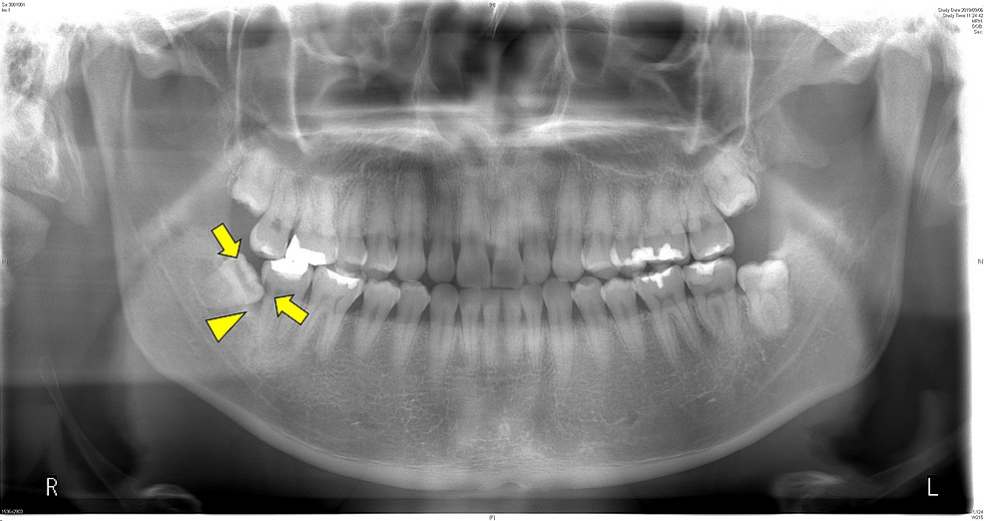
Panoramic radiograph showing a radiolucent lesion distal to the proximal surface of the second molar of the right mandible (yellow arrow) and pericoronitis around the periapical area of the third molar (arrowhead).
